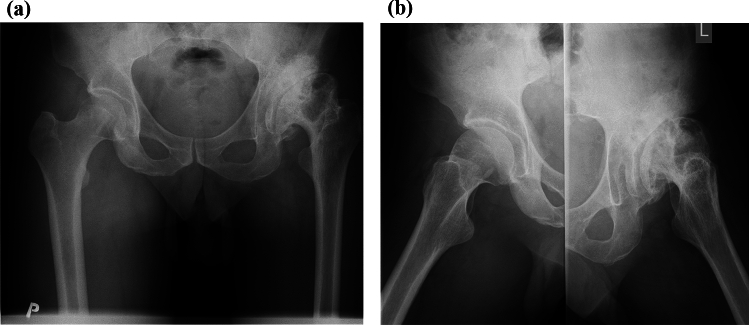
a Preoperative anteroposterior radiograph of 46-year-old patient with secondary postdysplastic coxarthrosis of the left hip joint (Crowe type II). The shortening of the affected extremity was of 2 cm. The deformity of the femoral head with the cysts is visible. b Preoperative axial radiograph of the same patient with postdysplastic coxarthrosis. The cyst in proximal part of the femoral head is visible
